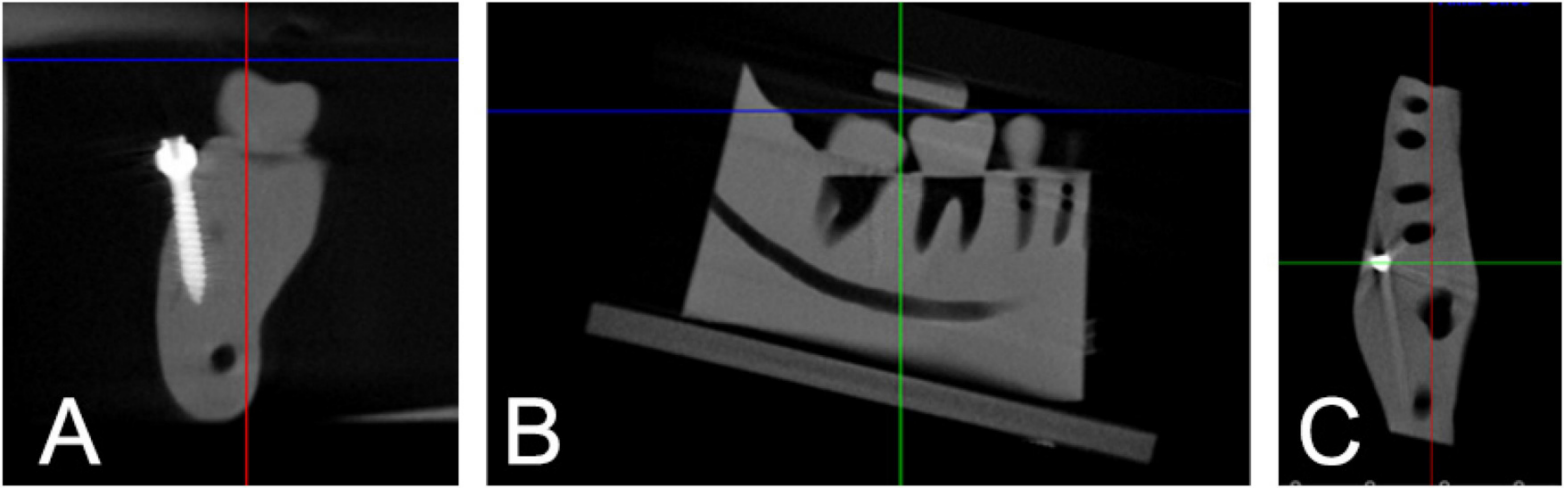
CBCT scans oriented three-dimensionally from (A) coronal, (B) sagittal, and (C) axial sections.
